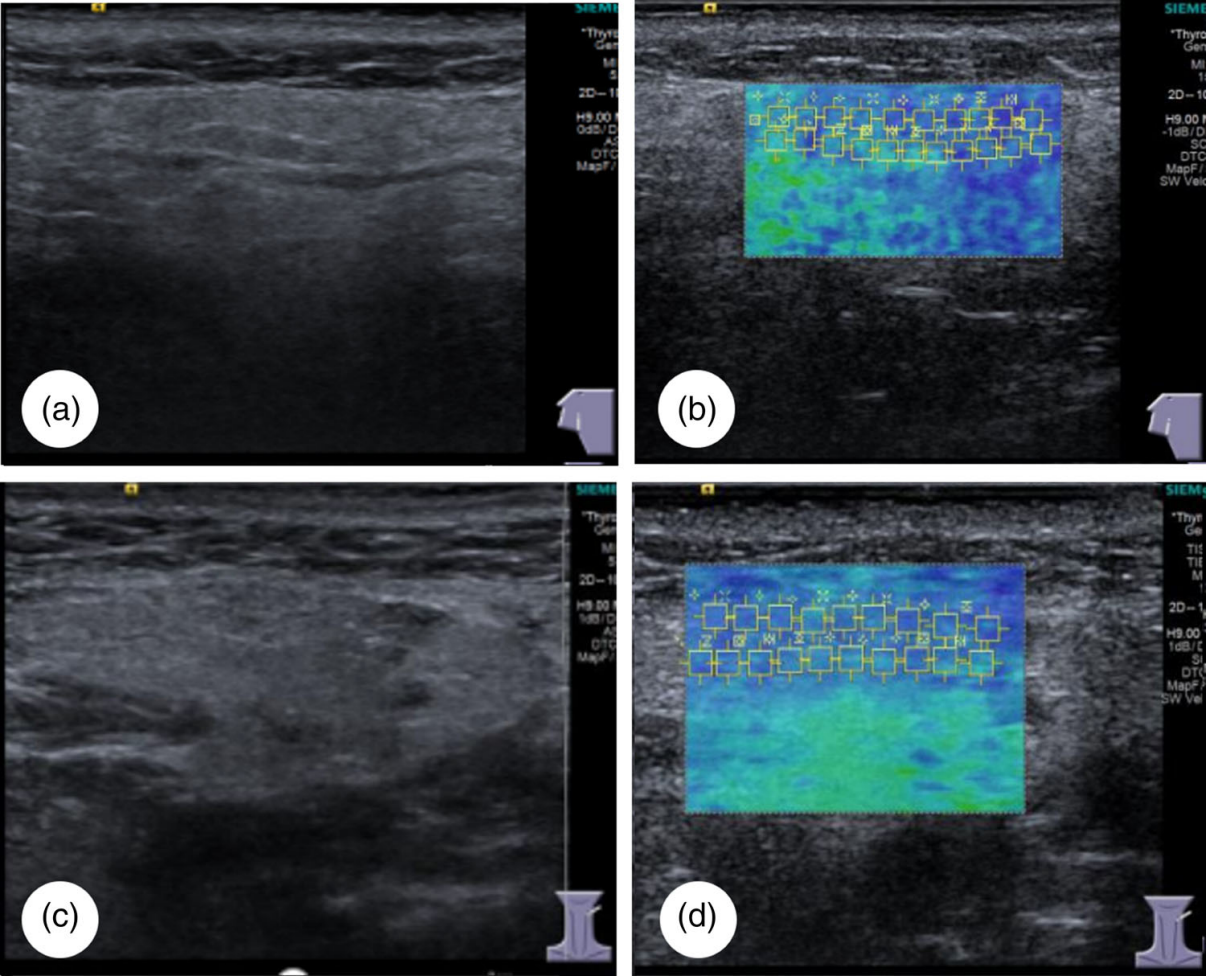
Ultrasound imaging and shear wave elastography of the parotid and submandibular glands in the control group. (a) Two‐dimensional ultrasonography of the parotid glands. (b) The shear wave velocity (SWV) of the parotid gland is 1.699 m/s. (c) Submandibular gland two‐dimensional ultrasonography. (d) The SWV of the submandibular gland is 1.696 m/s.
